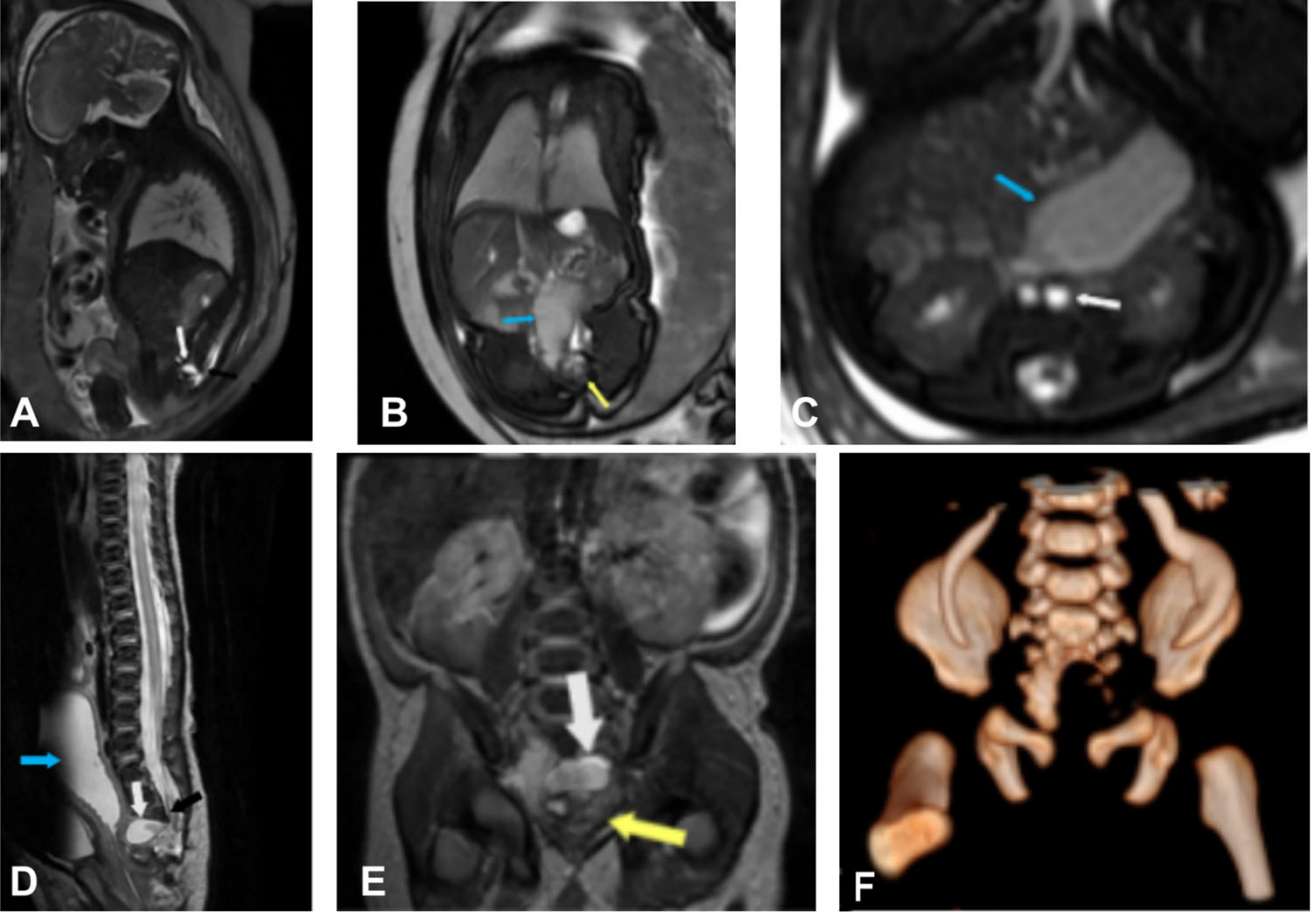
Currarino Triad. Gestational age: 33 weeks and 5 days. A Sagittal SS FSE T2. B Coronal FIESTA. C Axial FIESTA. Partial agenesis of the sacrum (black arrows), rectal dilation due to anorectal stenosis (blue arrows), anterior meningocele (white arrows), and presacral teratoma (yellow arrows). Postnatal control at 5 days of life (D) Sagittal FSE T2. E Coronal FSE T2. F VR reconstruction CT
